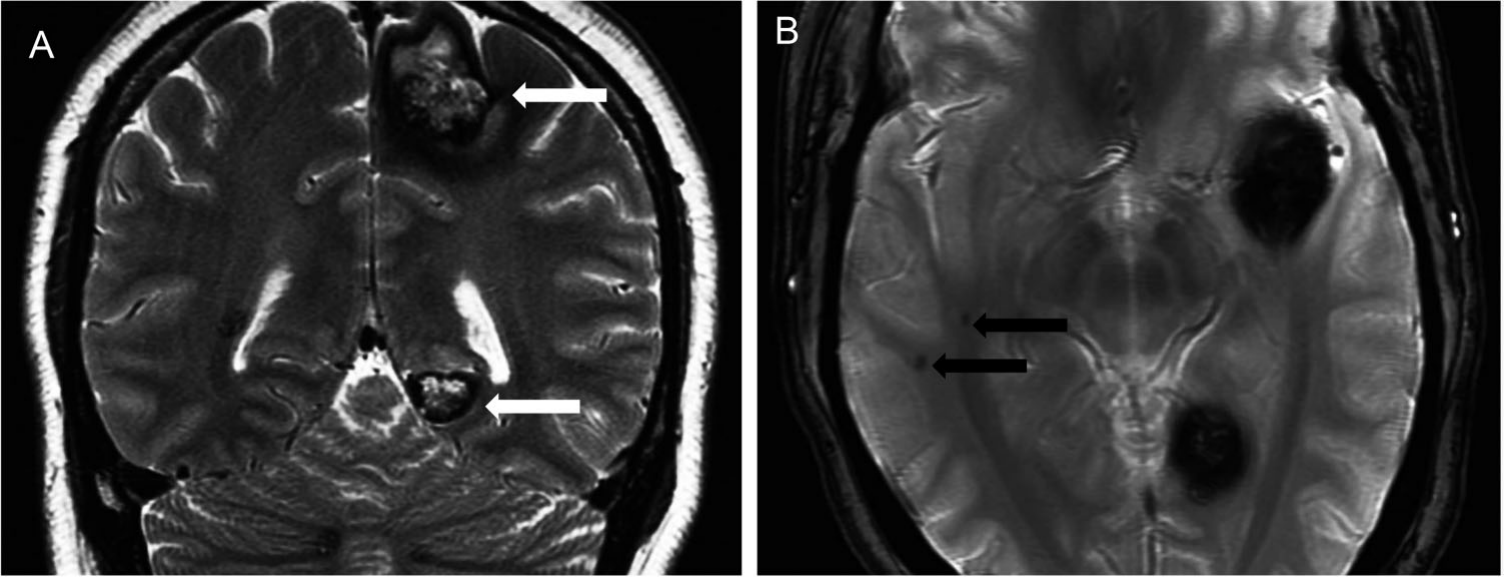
Familial cerebral cavernous malformations caused by a KRIT1 gene mutation in a 36-year-old woman with multiple epileptic foci (video-EEG) and chronic partial epilepsy. A) Coronal T2-weighted images show the typical appearance of a type 2 cavernoma (white arrows) characterized by mixed signal intensity with a hemosiderin ring. B) An axial T2*-weighted gradient-echo sequence revealing two type 4 cavernomas (black arrows) in the right temporal lobe, only observed using this sequence.
